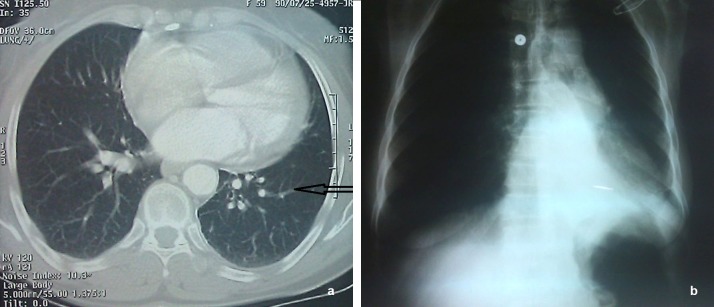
Chest X-ray, showing opacity in the left lung (a). Spiral computed tomography scan, demonstrating consolidation in the apical segment of the left lower lobe (b).
